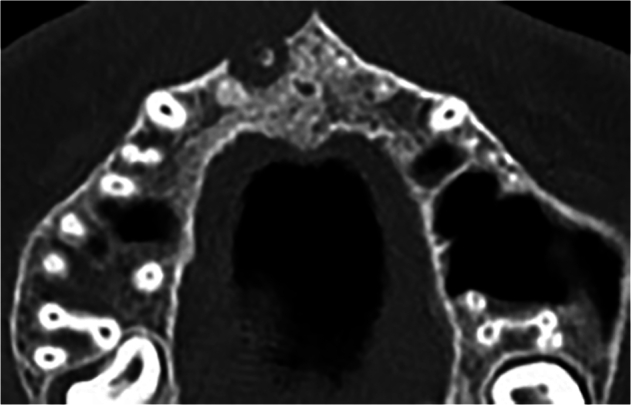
Preoperative CBCT image of the maxillary central incisor in axial view.
